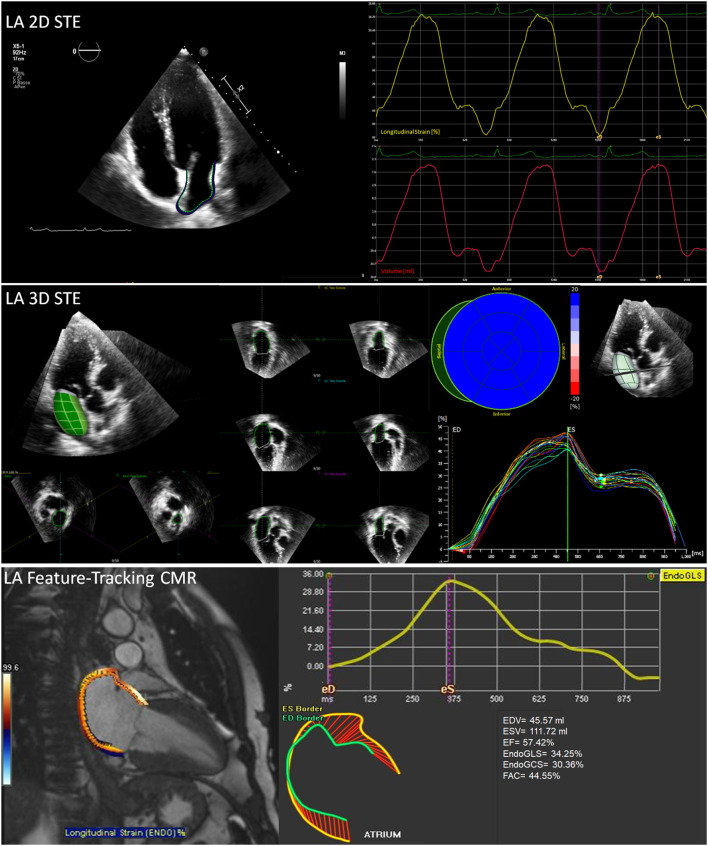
Non-invasive assessment of LA mechanics. Some elaboration and output examples of the mostly used imaging techniques are reported: (top) 2D speckle tracking echocardiography with atrial border contouring (top left), average longitudinal endocardial strain and volume changes (top right); (middle) 3D speckle tracking echocardiography with 3D LA volume reconstruction (middle left) and multi-segment longitudinal endocardial strain; (bottom) feature-tracking cardiovascular magnetic resonance (CMR) with atrial border contouring (left) and average longitudinal endocardial strain (bottom right). Abbreviations as in the text.
